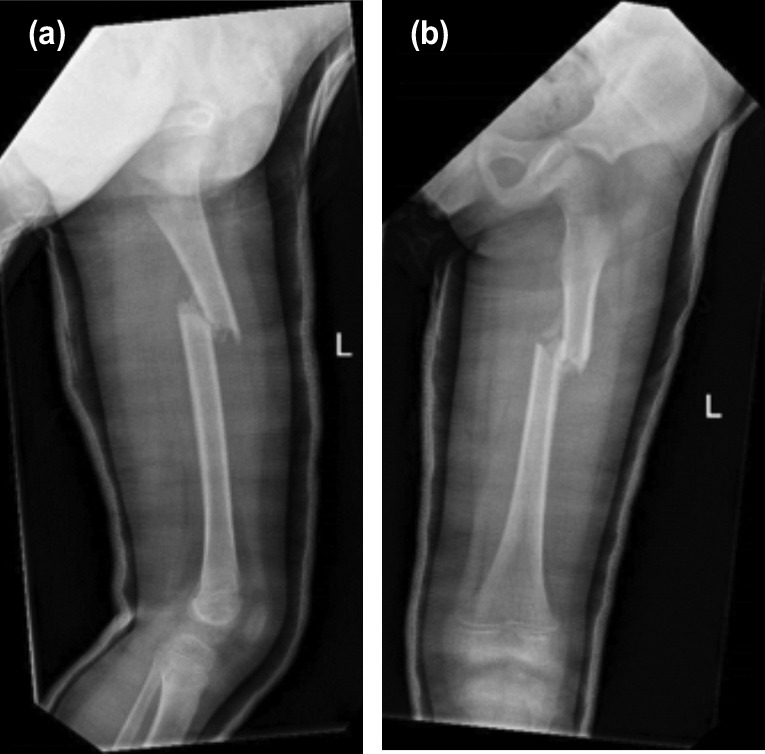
(a,b) Radiographs for the final shortening of patient B showing instability of the fracture.
